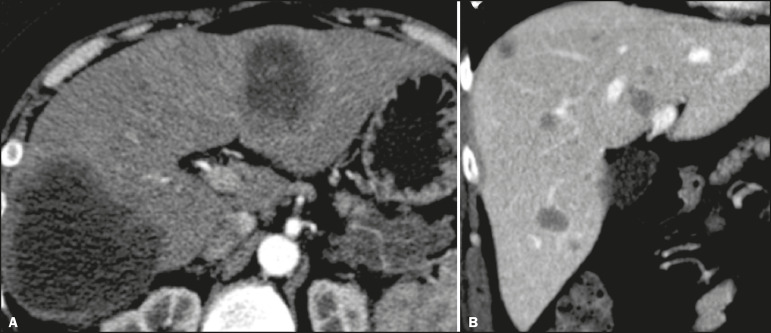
Arterial phase CT scan showing metastases from an NEN, characterized by masses (A) and multiple nodules (B) with a hypovascular enhancement pattern.
